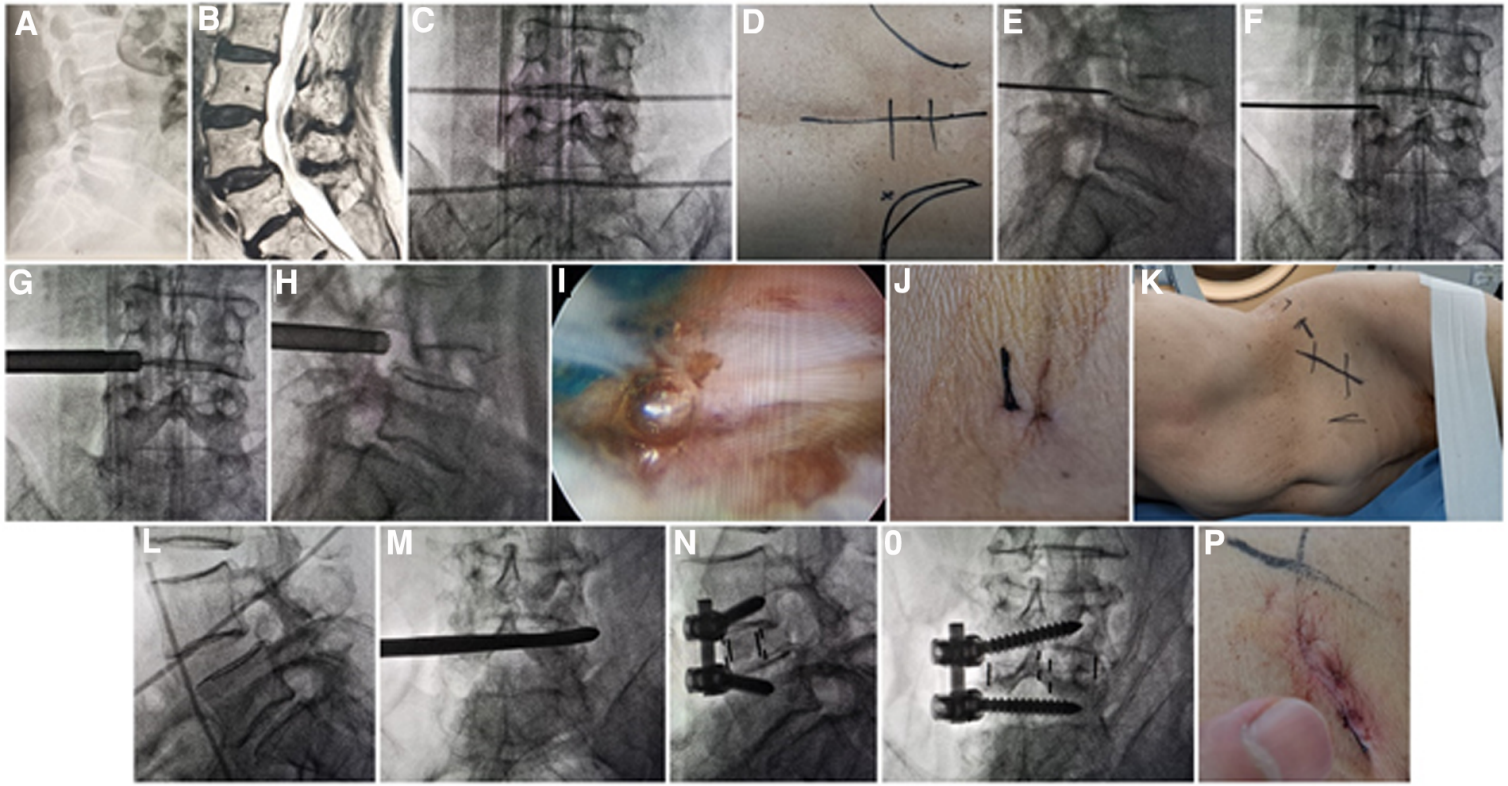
(A) X-ray and (B) MRI showed L4 Spondylolisthesis (I°) caused by degeneration in a 76-year-old female patient. Metal rods were placed transversely across the center of the target disc on (C) posteroanterior C-arm view to draw transverse lines. (D) The aimed reference point of puncture at surface was identified by the intersection of transverse line and longitudinal midline, and the entrance point of puncture (Gu's point) was located at the corner of flat back turning to lateral side. During puncture, once resistance disappeared, the C-arm view was taken to ensure that the tip of puncture needle was in the intracanal area close to the posterior wall of disc on (E) lateral x-ray and near the lateral border of pedicle on (F) posteroanterior x-ray. During press-down enlargement of foramen, when resistance disappeared, the tip of reamer should exceed the medial border of pedicle on (G) posteroanterior C-arm view and reach close to the posterior wall target disc on (H) lateral C-arm view. Under (I) endoscopic view, the compressed nerve root was freed after the hypertrophic ligamentum flavum and herniated disc were removed. (J) The stab incision of about 8 mm for PTES was closed. (K) Patient was placed into right decubitus position for mini-incision OLIF and anterolateral screws rod fixation. The anterior line of L4/5 intervertebral space was positioned using (L) C-arm view. The spatula was inserted into L4/5 intervertebral space to cut the contralateral fibrous annulus, which was confirmed by (M) x-ray image. After the OLIF cage was placed into disc space parallel to the endplate and anterolateral screws rod fixation was performed, (N) lateral and (O) posteroanterior C-arm view confirmed good position of internal instruments. (P) The mini-incision of OLIF was sutured finally. PTES, percutaneous transforaminal endoscopic surgery; OLIF, oblique lumbar interbody fusion.
